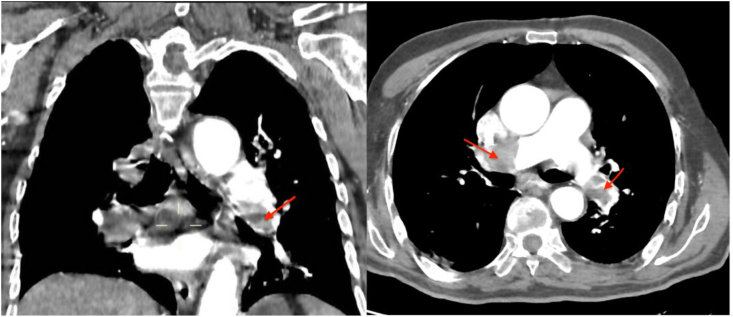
A: Axial image from the CT pulmonary angiogram (CTPA) demonstrates bilateral proximal pulmonary emboli B: Coronal image from the CT pulmonary angiogram.
